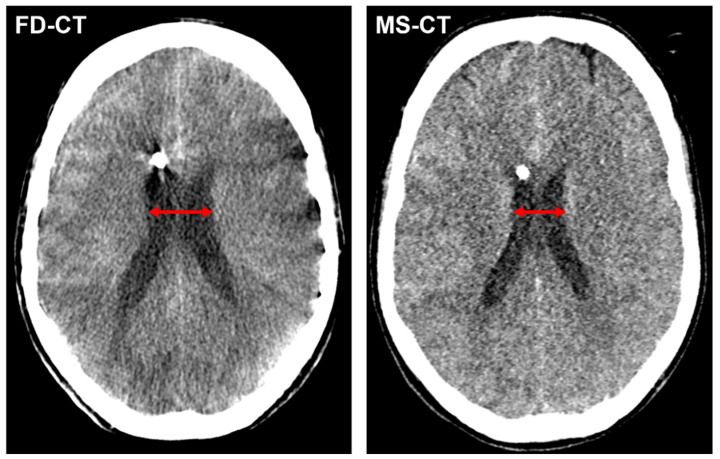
Exemplary cella media distance (CMD) measurement (red arrow) in a FD-CT and MS-CT dataset of a patient with endovascular treated, aneurysmal SAH and slightly declining width of the lateral ventricles from postinterventional FD-CT to MS-CT.
